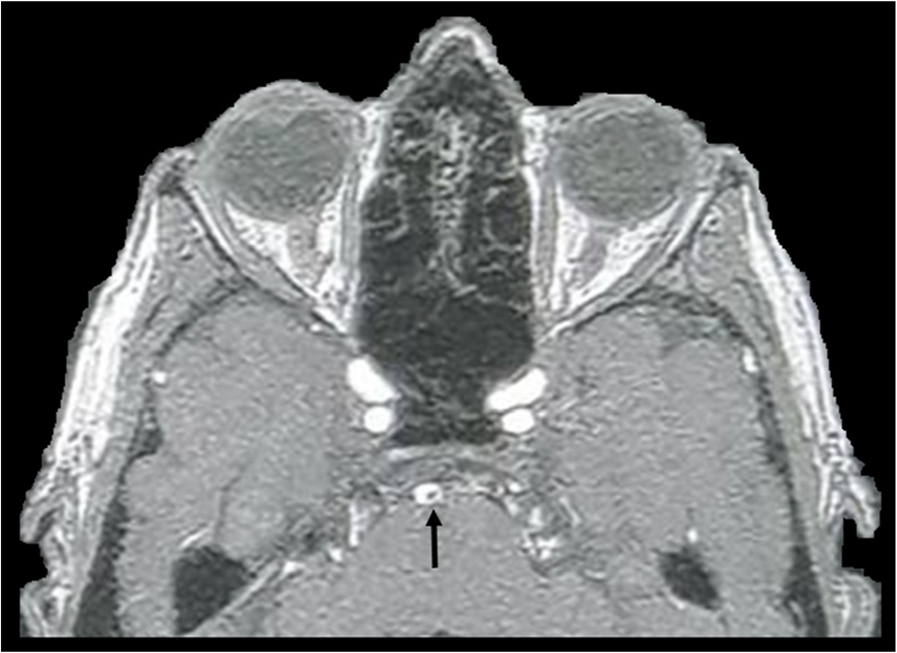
Axial CT intracranial angiogram demonstrates a filling defect within the basilar artery (black arrow) in a patient with myelofibrosis. Other multiple filling defects were seen in both vertebral arteries (not shown). Arterial embolisms are common in myelofibrosis and can result in transient ischaemic events, cerebrovascular accidents, angina or myocardial infarctions, and peripheral vascular disease
